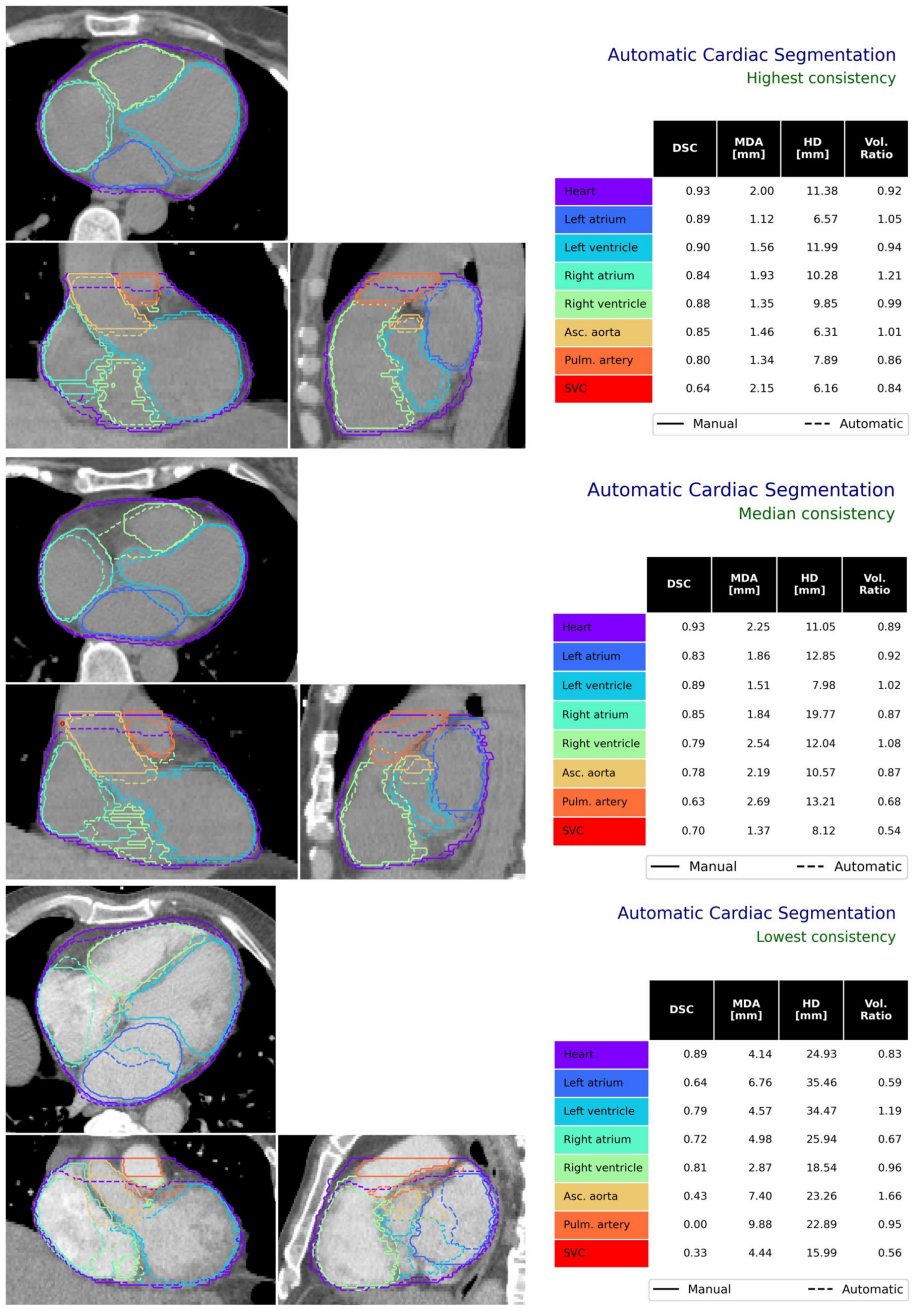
Orthogonal slices for three patient CT scans demonstrating the performance of the proposed automatic segmentation method. These are shown for patient for which the automatic delineations had the highest (top), median (middle) and lowest (bottom) consistency with manual contours. Acronyms: SVC - superior vena cava, DSC - Dice Similarity Coefficient, MDA - mean distance to agreement, HD - Hausdorff distance, Vol. - volume
